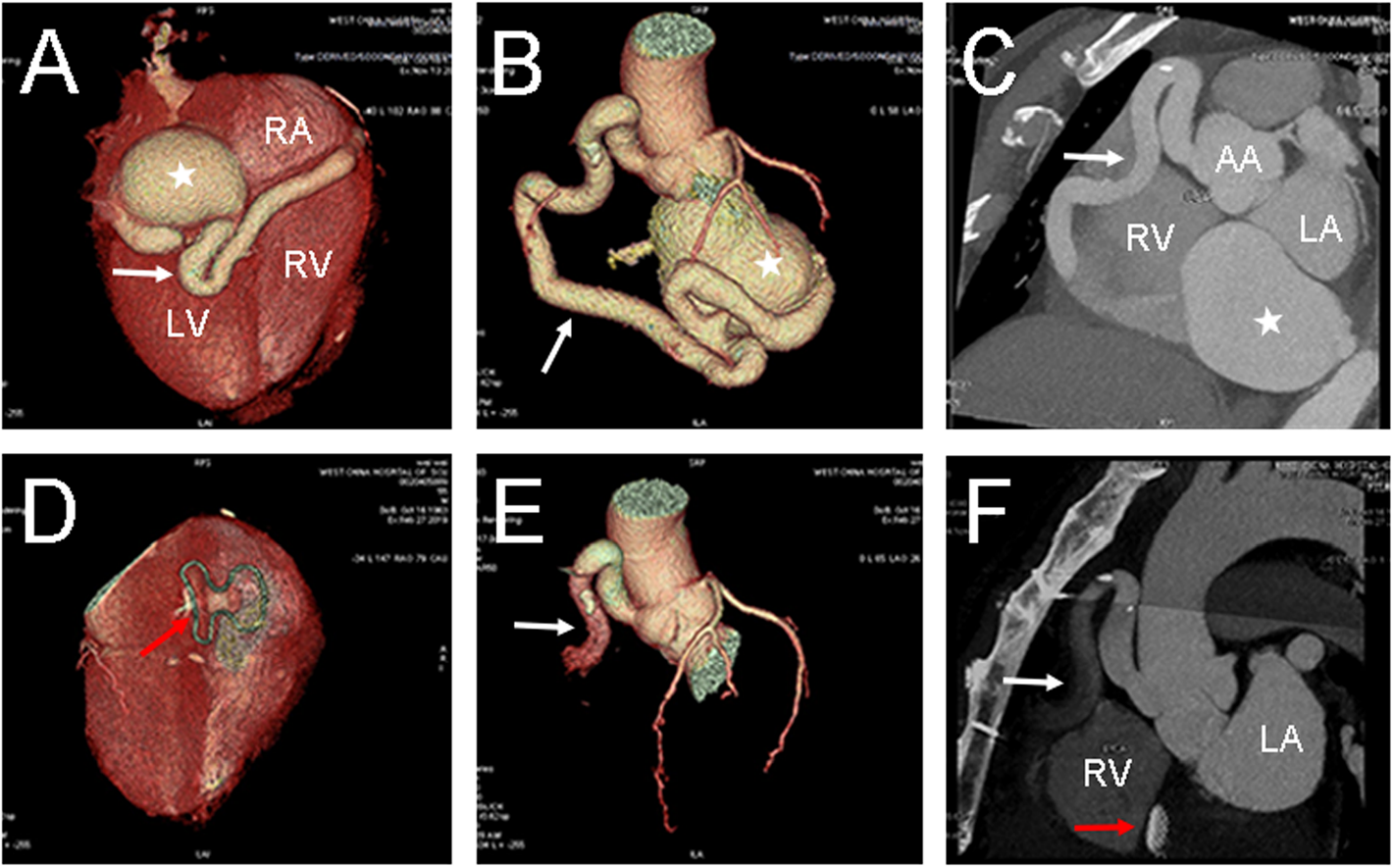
Preoperative and Postoperative CT findings: a, b, c Pre-operation CT showed tortuous and dilated RCA (white arrow) and the diameter increased to 11 mm, a giant aneurysm (65* 48 mm) (white star) originating from the distal RCA and draining into the RA; d, e, f In the third postoperative months, there was no displacement of the occluder (red arrow) and the thrombus formed at the distal end of the right coronary artery was undeveloped.(RCA right coronary artery; LA left atrium; RA right atrium; RV right ventricle; AA ascending aorta)
